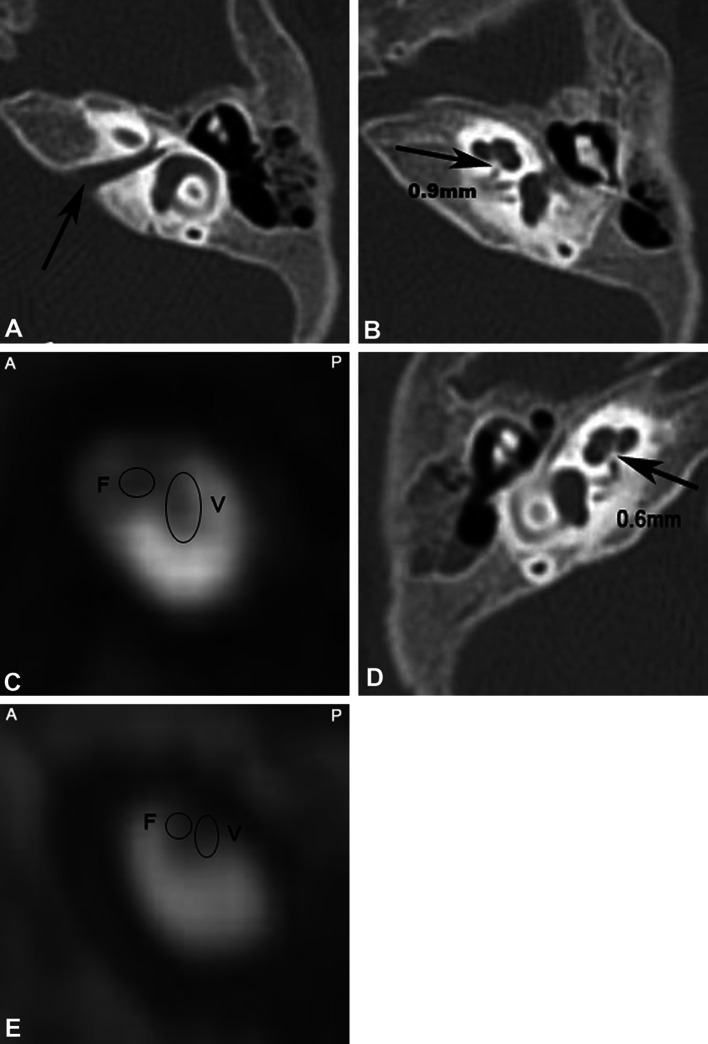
Stenosis of bilateral IAMs with an undetected CN. Male, 4 years old. Total hearing loss in the right ear, moderately severe hearing loss in the left ear. a, b, d CT shows bilateral stenosis of IAM, normal development of the cochlea (*arrow*). The diameters of the CNC are 0.9 and 0.6 mm. c, e Undetected CN with oblique sagittal reconstruction of MRI
